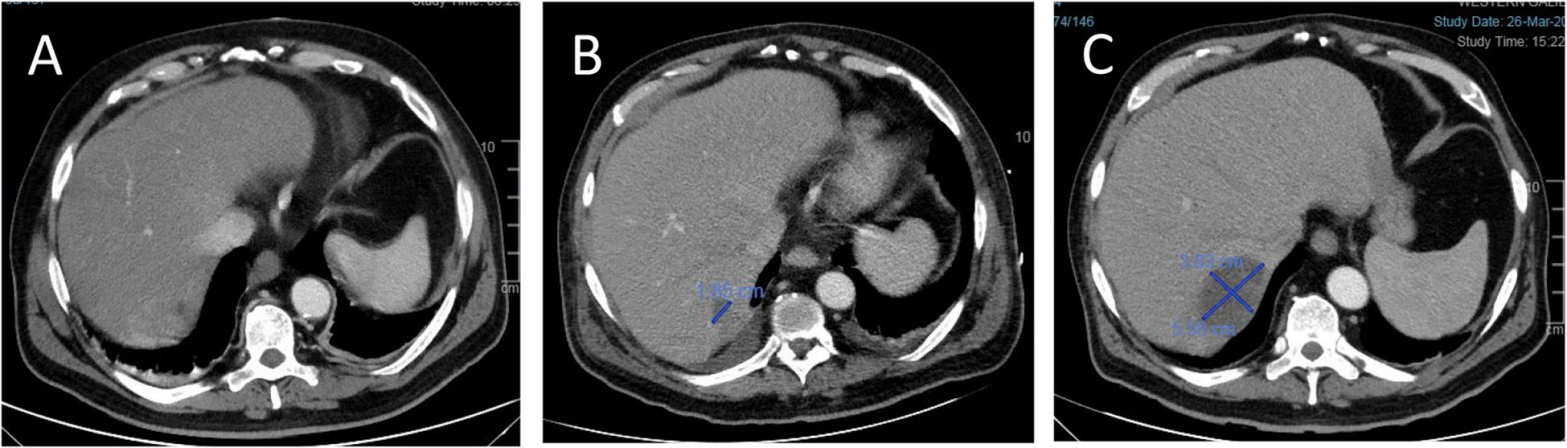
Liver abscess development on consecutive double contrast abdominal computed tomography scans. Scans were done on days 3 (a) 7 (b) and 26 (c) post-endoscopic retrograde cholangiopancreatography
